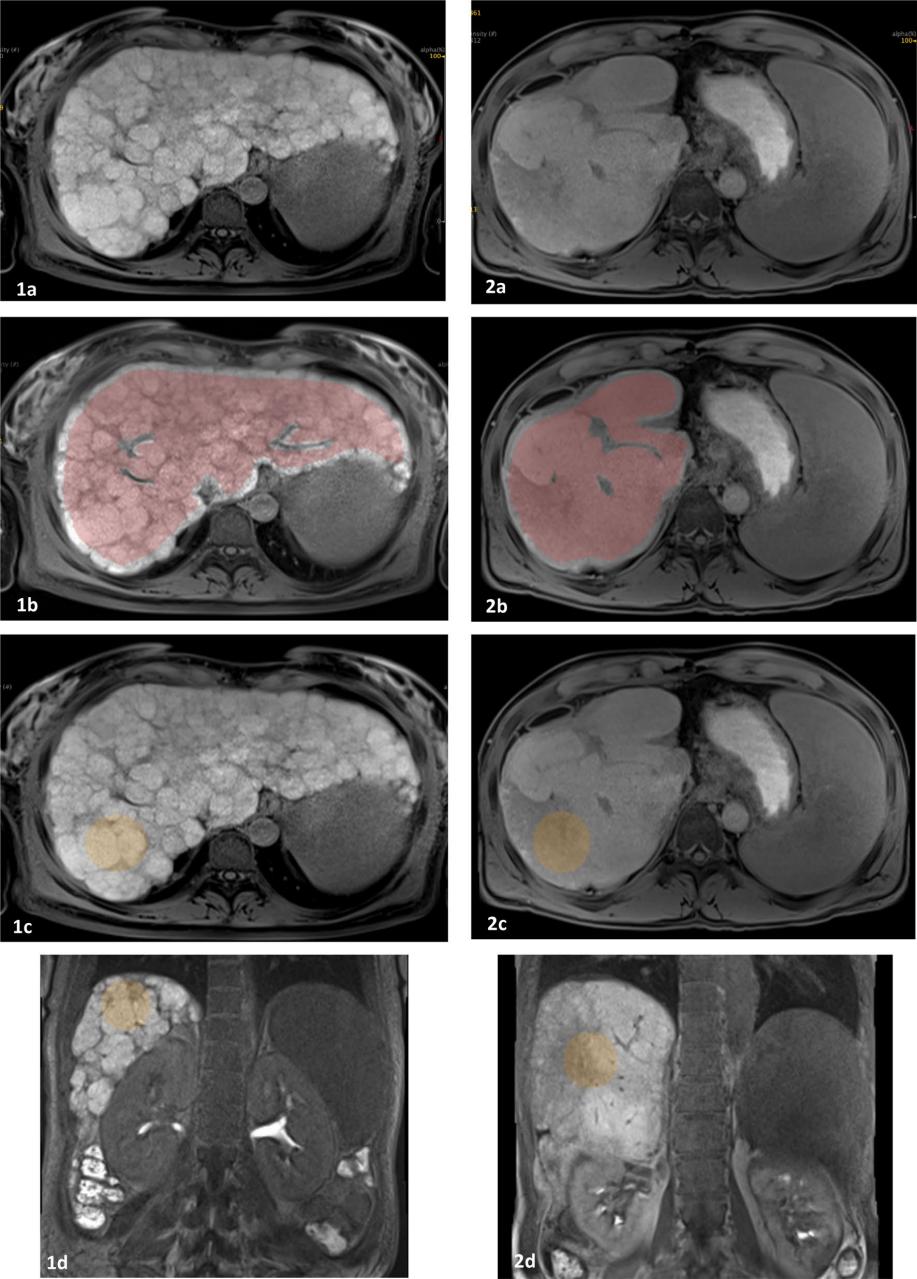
Gadoxetic acid-enhanced hepatobiliary phase (HBP) MR images showing region of interest (ROI) segmentation in two-dimensional (2D) and three-dimensional (3D) format. HBP images, axial before (a) and after (b,c) 2D (b) and 3D (c) ROI segmentation as well as coronal (d) reconstructed images showing 3D ROI segmentation. Patient 1 is a 36-year-old female with nonalcoholic steatohepatitis (NASH)-associated liver cirrhosis. Patient 2 is a 47-year-old male with primary sclerosing cholangitis complicated by liver cirrhosis.
